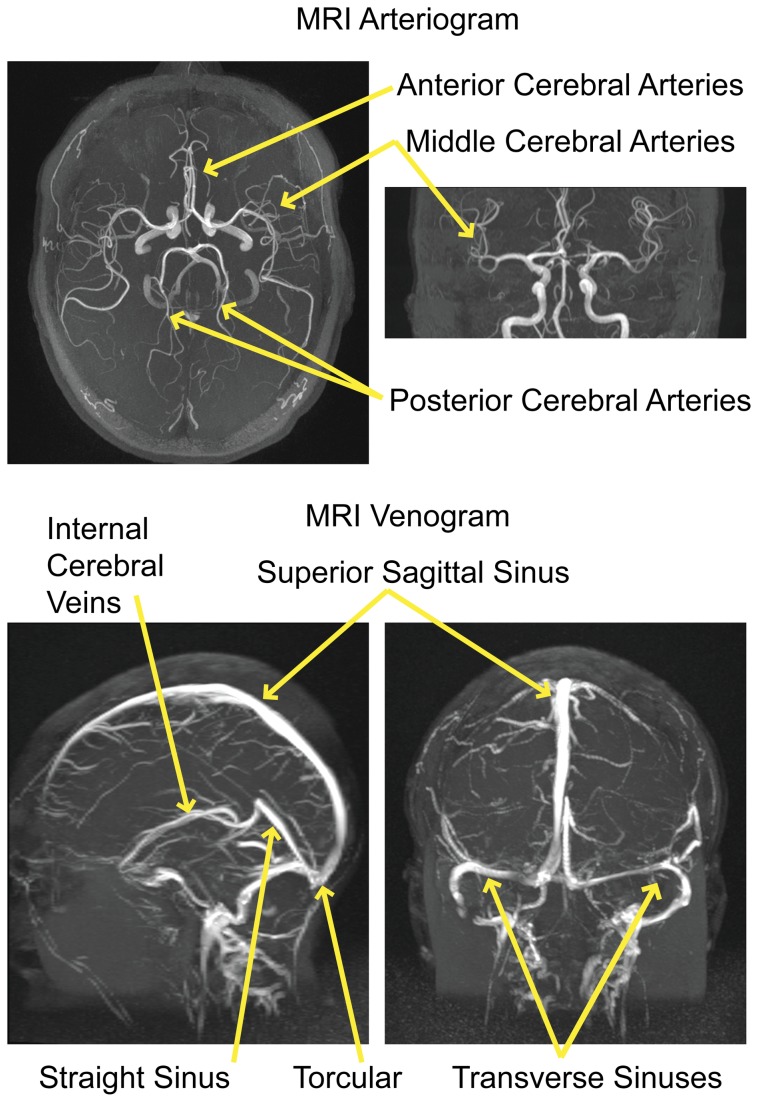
Representative MRI arteriogram (above) and MRI venogram (below) from one subject, with major arteries and venous sinuses labeled, shown in maximum intensity projection for axial and coronal views.
